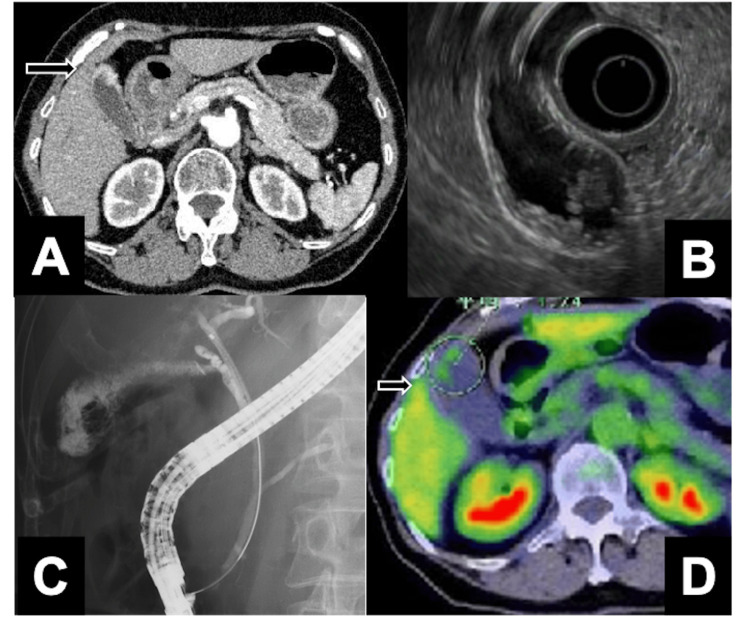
Preoperative imaging findings A: Contrast-enhanced CT revealed wall thickening at the fundus of the gallbladder (arrow), raising suspicion of gallbladder carcinoma. B: Endoscopic ultrasonography (EUS) suggested gallbladder carcinoma. C: Endoscopic retrograde cholangiopancreatography (ERCP) demonstrated a pancreaticobiliary maljunction without significant dilatation of the common bile duct. The amylase level of the bile juice collected during the ERCP was 200,111 IU/L. D: Positron emission tomography with fluorodeoxyglucose (FDG-PET) demonstrated mild FDG uptake in the tumor (arrow).
